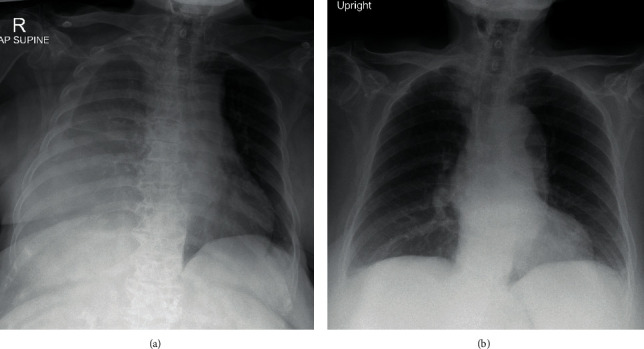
Chest X-rays revealing moderate amount of right pleural effusion with pressure effect caused trachea shift to the left (a) and complete resolution of right pleural effusion after discontinuing lenvatinib for 1 week (b).
